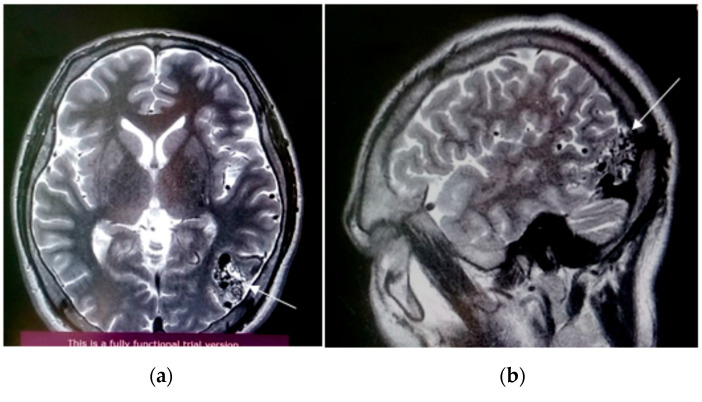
Axial (a) and sagittal (b) views of MRI obtained at initial presentation of the patient. AVM in the middle cerebral artery (MCA) and hypoplasia of cerebellar tonsils with subarachnoid hemorrhage (SAH). Right sided sinusitis and ethmoiditis. White arrows indicate location of AVM on the MRI.
